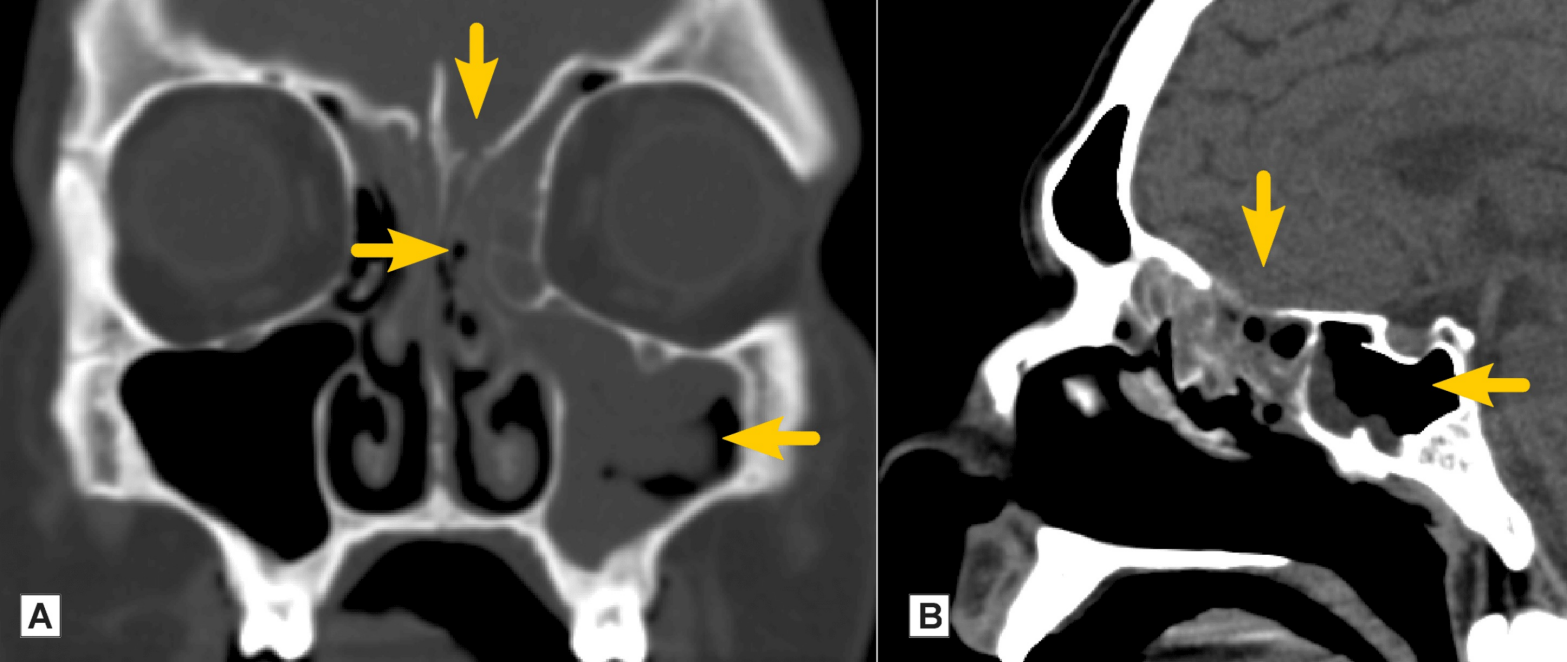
Native CT evaluation (Case 1) A: coronal plane showing erosion of lamina cribrosa and left-sided pansinusitis (arrows); B: sagittal plane showing routh to the anterior cranial fossa and sphenoidal sinusitis (arrows)
